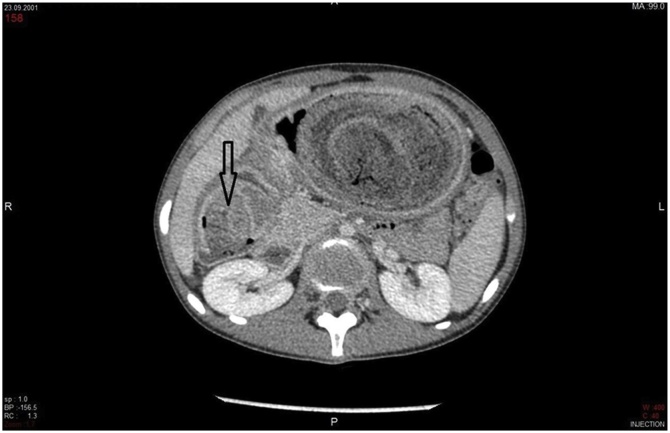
Contrast enhanced CT, sagittal view at the level of pyloric canal: passage of the large mass lesion is noted through the pyloric canal (Open arrow to the duodenum (D)).
